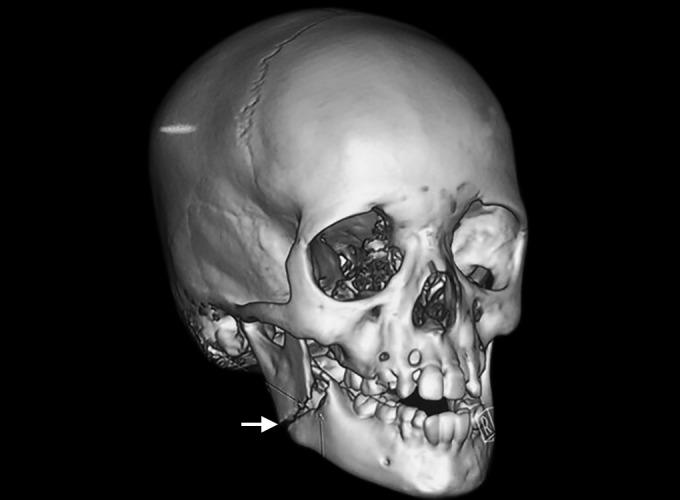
Three dimensional facial CT (arrowhead) with fractured segments
